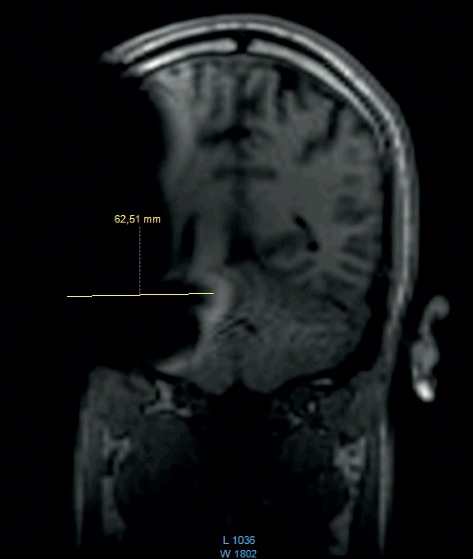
MRI survey pattern of the coronal plain of a 1.5 T scanning of an Advanced Bionics 3D Implant.
